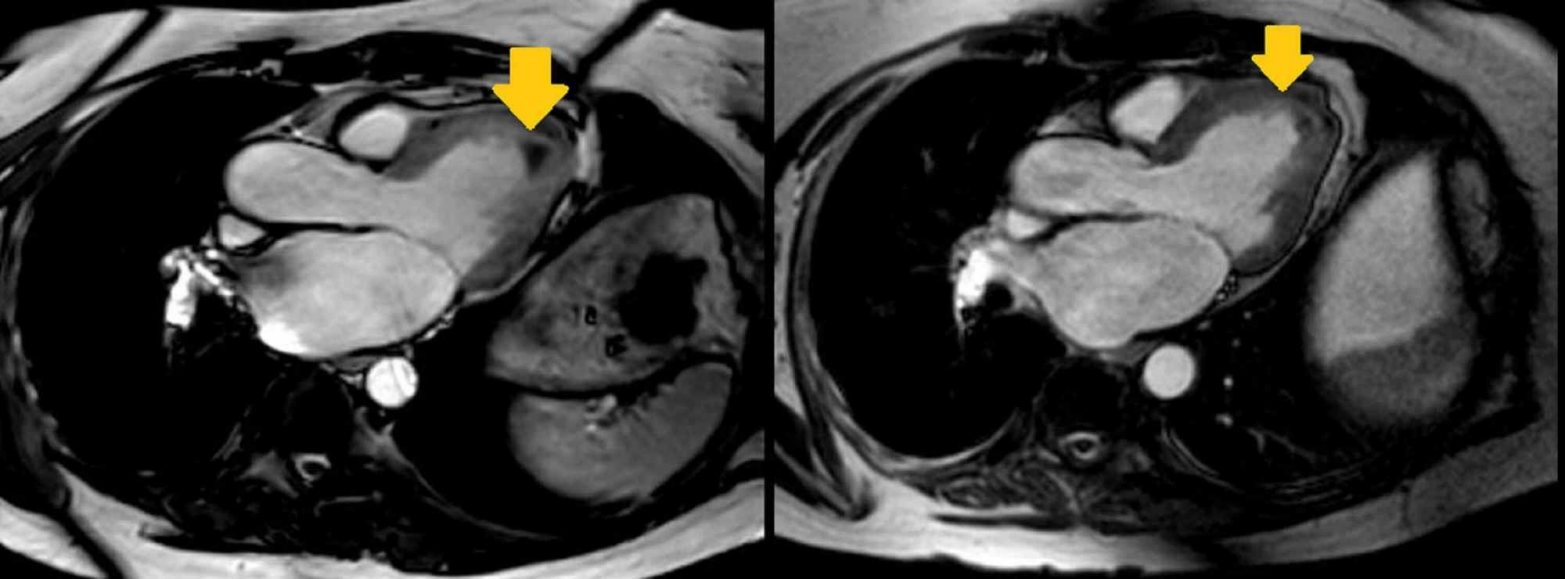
Cardiac MRI (before and after) Cardiac MRI four months later showing improvement in the hypoattenuation of the left ventricular apex (arrows indicating comparison)
